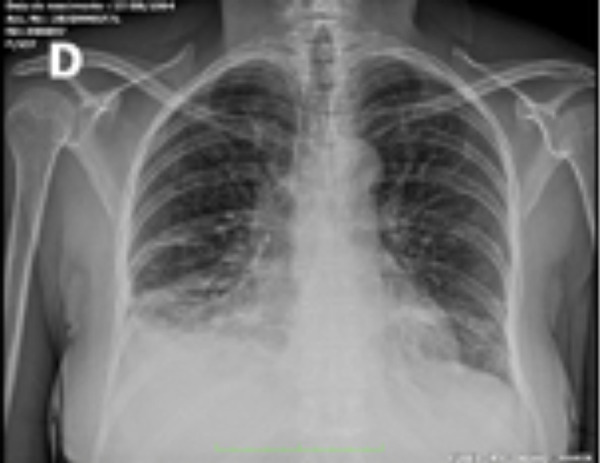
Anteroposterior chest radiograph.
